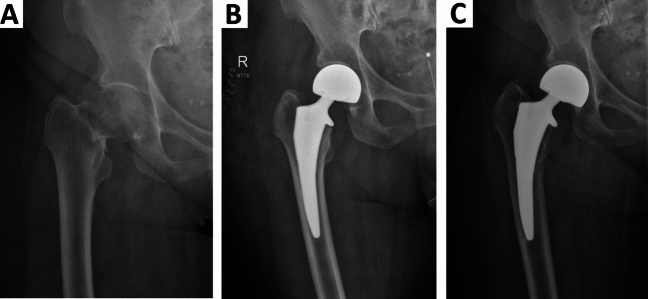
(a) X-rays showing a case of femoral neck fracture in an 88-year-old woman, (b) treated with Actis® stem HA 1 week and (c) 2,7 years following surgery. Successful, complication-free clinical follow-up and radiological osteointegration was observed in this case. HA, Hemiarthroplasty
